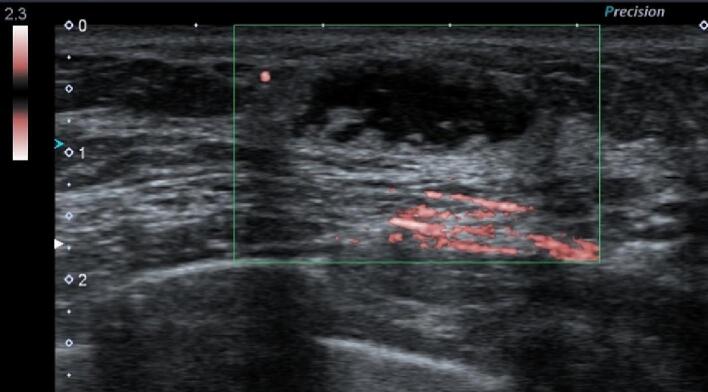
The breast ultrasound shows an oval hypoechoic mass accompanied by the front and lateral boundaries that are partially obscured. Internal heterogeneity and posterior echo enhancement are also revealed.
